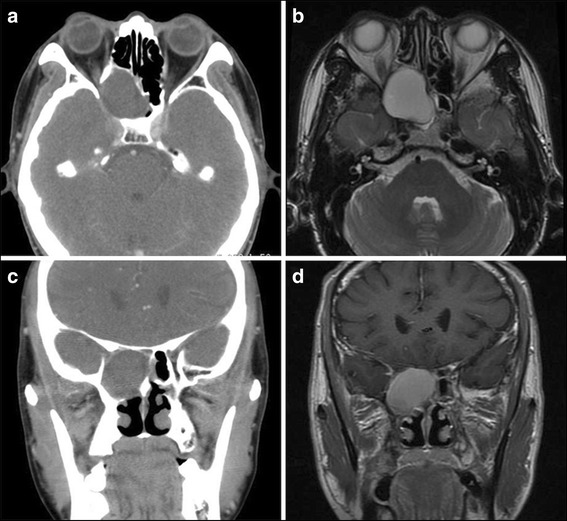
Case 5, male, 51y, sphenoid sinus mucocele (right, VA = 30/200). Neuroimaging showed a hemispherical abnormal mass rose from right sphenoid sinus with expansion to surrounding areas. There is extension into the right orbital apex and compression of the right optic nerve. a: Axial CT scan showing the expansile lesion. b: Axial, T2-weighted MRI scans with homogenous, fluid density lesion in the right sphenoid sinus. c: Coronal CT scan shows chronic bony expansion of the sinus. d: Coronal, T1-weighted, contrast-enhanced MRI scan demonstrating the fluid-filled lesion
